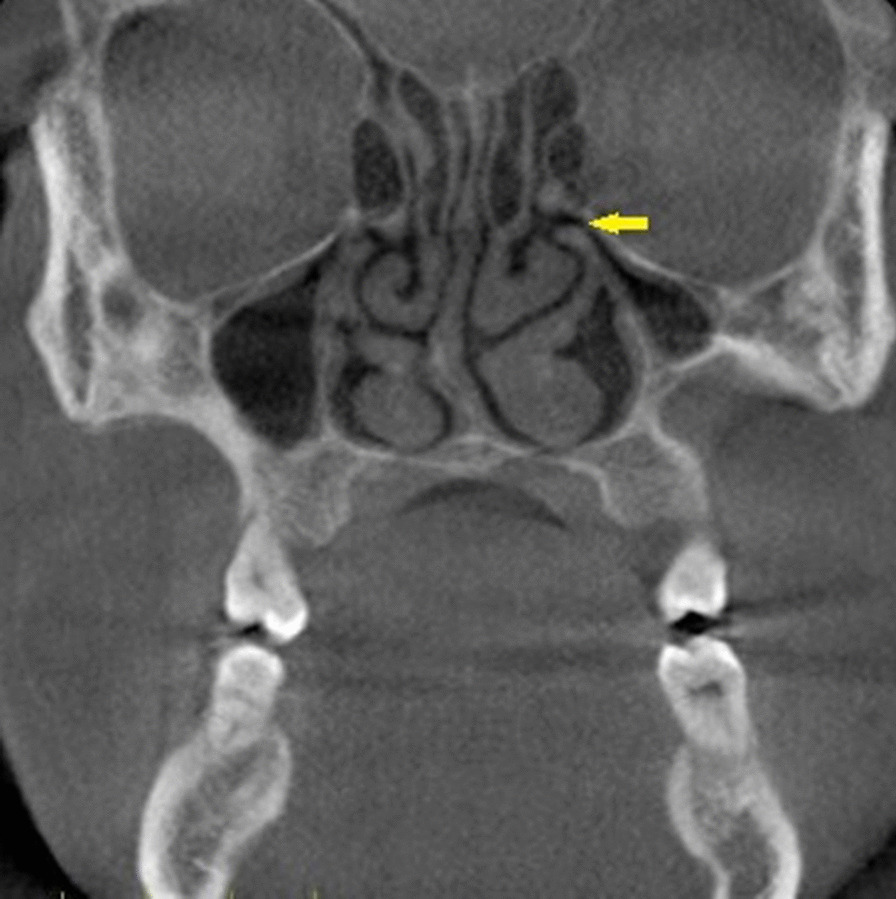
Coronal CBCT section showing the site (ostium and uncinated process) at which the middle width of the turbinate was determined
